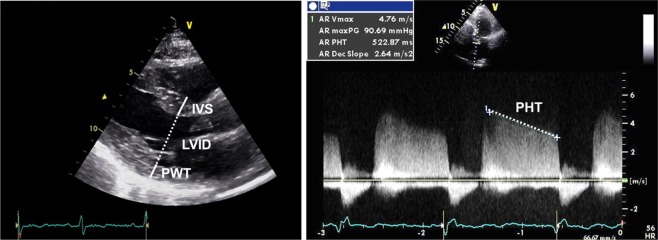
Echocardiographic parasternal long-axis view with linear measurements of the left ventricle performed at end-diastole. Left ventricular mass was calculated by the formula: LV mass = 0.8*1.04*[(IVS + LVID + PWT)³−LVID³] + 0.6 g where IVS is interventricular septum, LVID is LV internal diameter, and PWT is inferolateral wall thickness (left panel). Pressure half-time (PHT) was measured by analysis of the continuous-wave Doppler velocity curve of aortic regurgitation jet (right panel).
